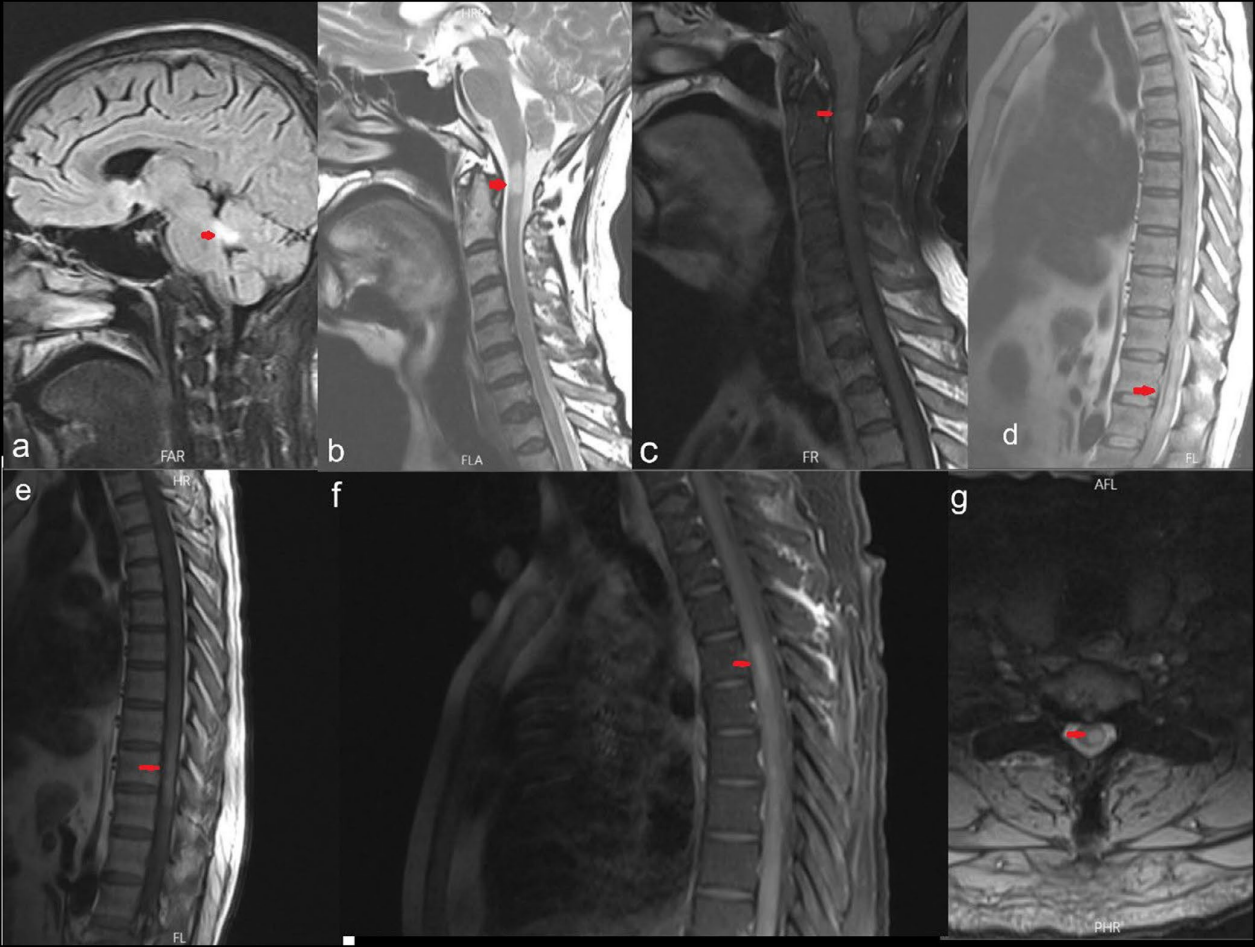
(a) Brain magnetic resonance imaging revealed a third ventricle peri‐ependymal lesion as well as cerebellar peduncle involvement. (b, c) Cervical cord involvement with enhancement. (d) Thoracic MRI revealed hyperintense longitudinal lesion in T2. (e) Imaging showed hyperintensity in T1 which meant hemorrhagic myelitis. (f) Imaging with enhancement. (g) Centrally located hyperintensity has been shown in the axial view of the thoracic cord. Arrows represent the lesions.
